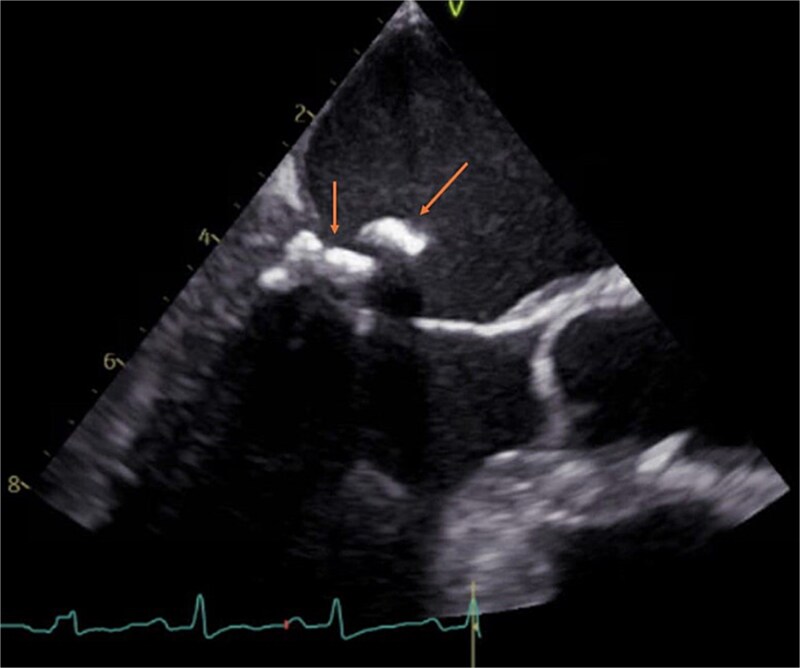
Transesophageal echocardiography (TEE). Mid-esophageal long-axis view demonstrating degenerative mitral valve disease with two mobile, calcified masses (arrows) attached to the posterior leaflet. The larger mass (1.4 × 1.0 cm) is attached to the P2 scallop, while a smaller mass (1.0 × 0.5 cm) is attached to the P1 scallop.
